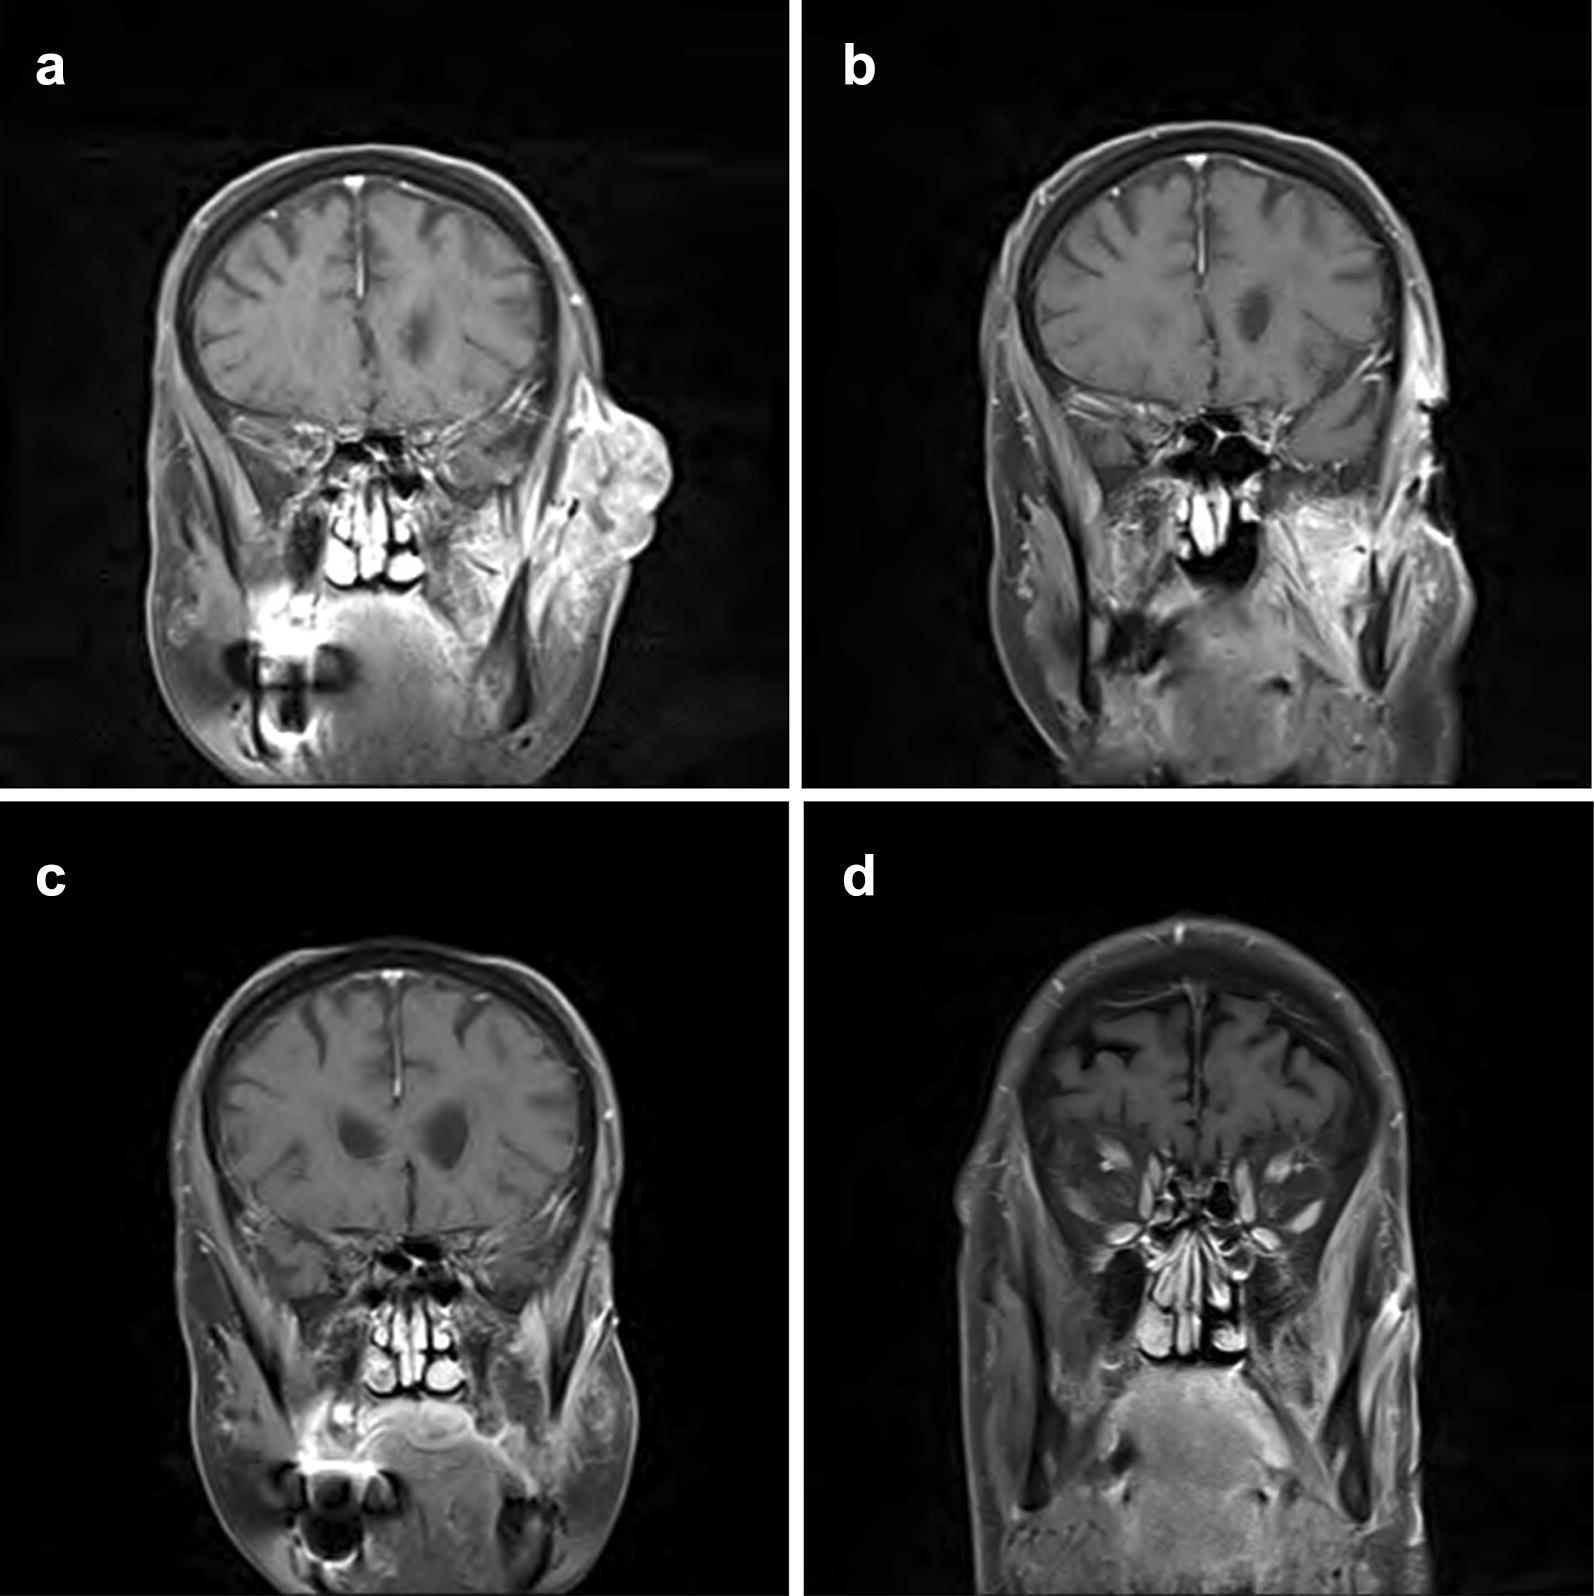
a–d Coronal T1+ Gadolinium MRI demonstrating 3.2 × 3.8 × 4.8 cm heterogeneous mass and progressive direct bone invasion extending to the junction of the left temporal bone and zygomatic arch. There is involvement of the deep left parotid gland at the level of the facial nerve with evidence of perineural extension along the V3 nerve to the foramen ovale. Figure 1b through 1d demonstrate dramatic improvement in the patient’s tumor burden
